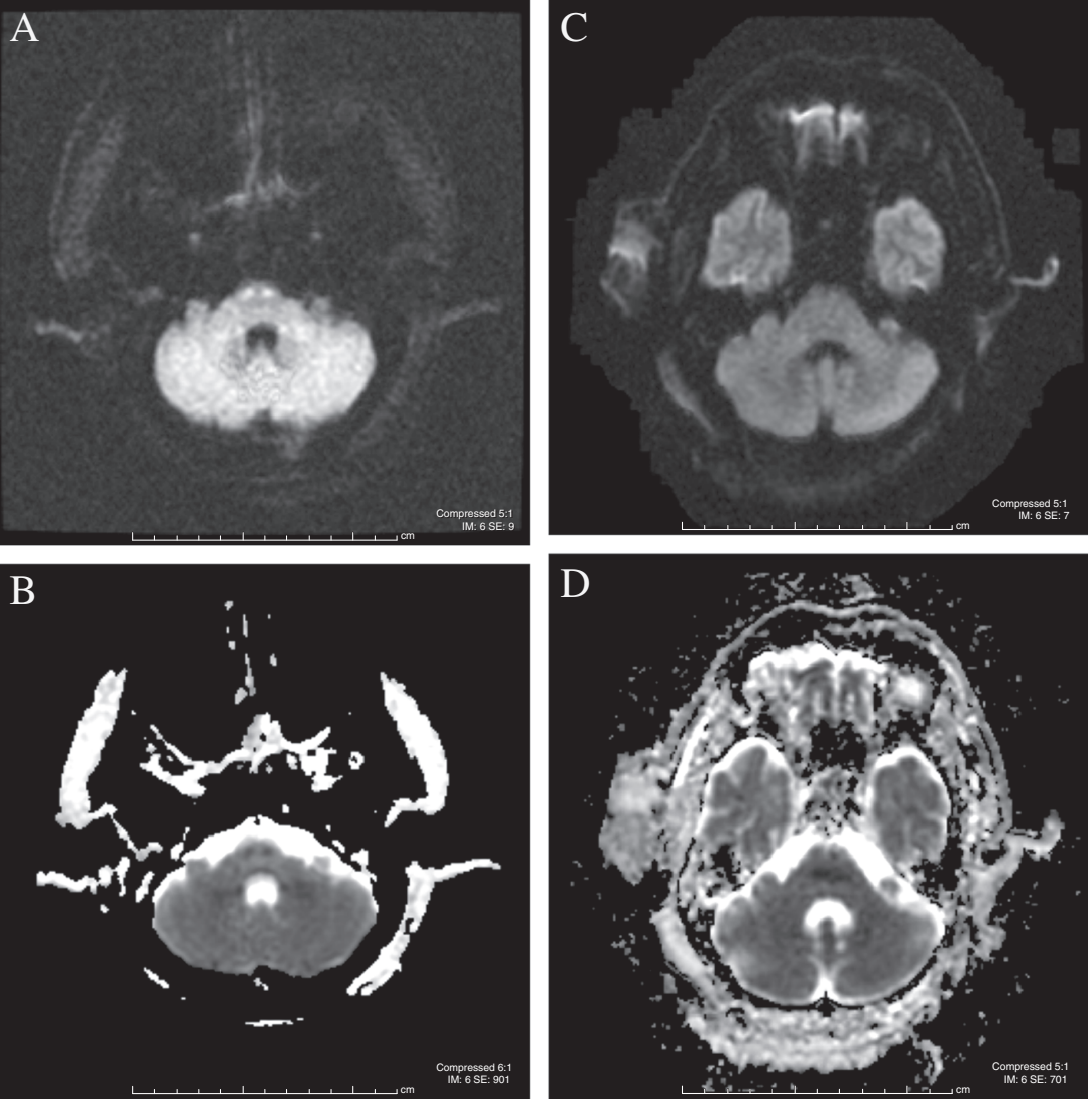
Magnetic resonance imaging of the brain demonstrating restricted diffusion in the bilateral medullary pyramids and pons. (A) Diffusion-weighted image. (B) Apparent diffusion coefficient image. Repeat magnetic resonance imaging of the brain performed two months later demonstrates resolution of the diffusion restriction: (C) diffusion-weighted image; (D) apparent diffusion coefficient image.
